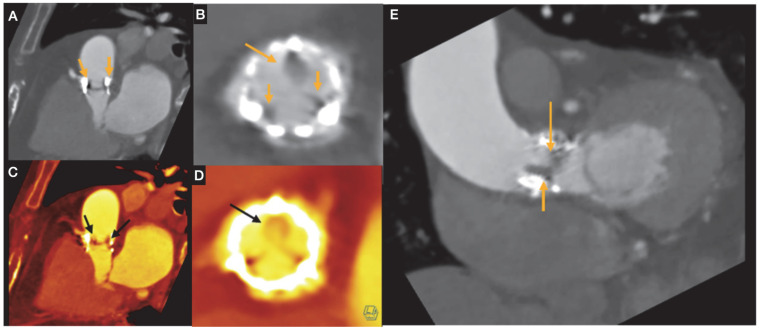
An 84 year old woman with a history of TAVI. She presents with dyspnea. Cardiac CT angiography revealed an aortic valve prosthesis with evidence of hypoattenuation and leaflet thickening (HALT), and absence of complete coaptation, with a calculated aortic valve orifice (AVo) area of 1.1 cm^2^ (prosthetic valve dysfunction). Top images (A,B) show the valve stent and thickened leaflet in an oblique view (A), and a short-axis view of the valvular structure during systole (B), with arrows indicating areas of hypoattenuation consistent with HALT. Bottom images (C,D) display color-coded reconstructions to enhance structural contrast. Arrows highlight the valvular stent. The bottom-right image (D) demonstrates lack of leaflet coaptation, contributing to valvular regurgitation (arrow). (E) depicts an oblique view of the valve during systole, showing absence of excursion of one of the leaflets as compared to the other (arrows).
